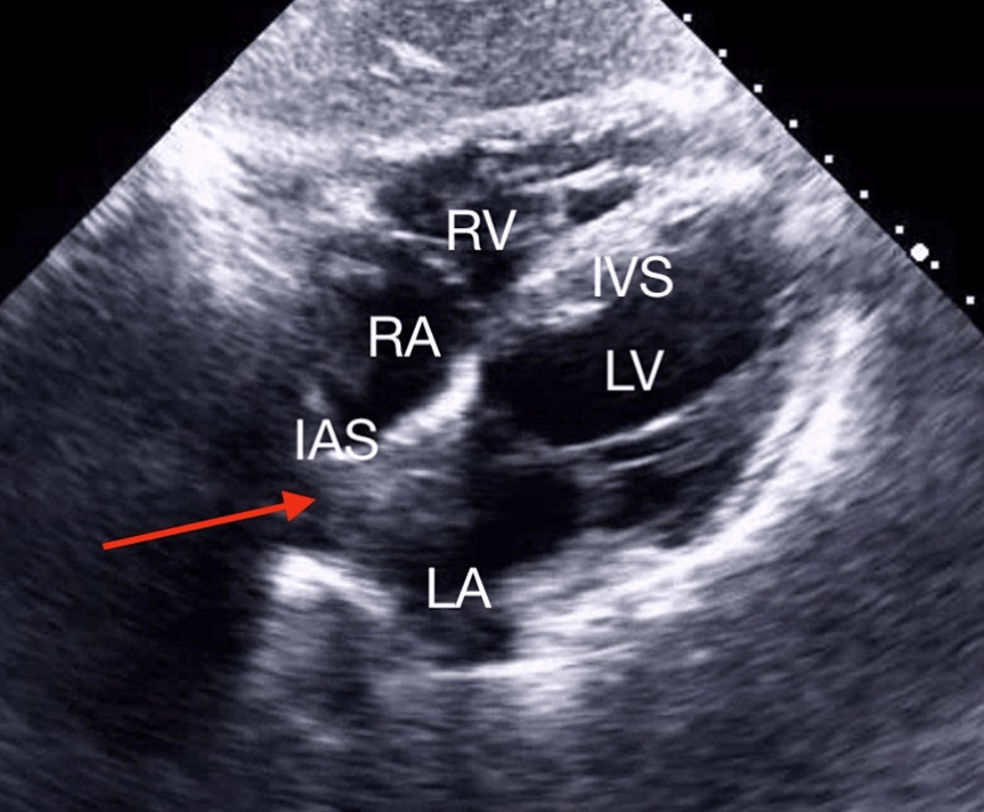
Subcostal Chamber View of the Atrial Myxoma Transthoracic echocardiogram showing mass (arrow) protruding into left atrium causing partial obstruction during systole. RA = right atrium; LA = left atrium; RV = right ventricle; LV = left ventricle; IAS = interatrial septum; IVS = interventricular septum
